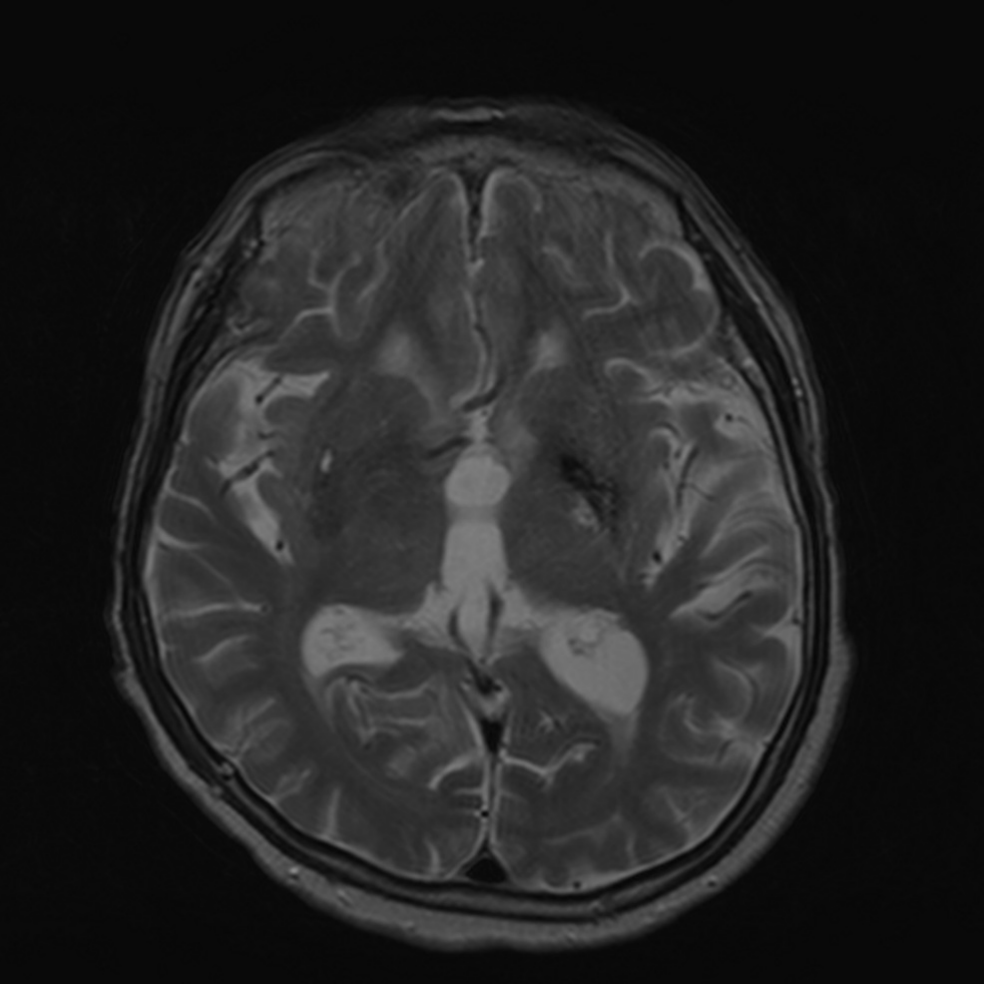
T2 weighted MRI showing evidence of prior hemorrhage in the left basal ganglia.
